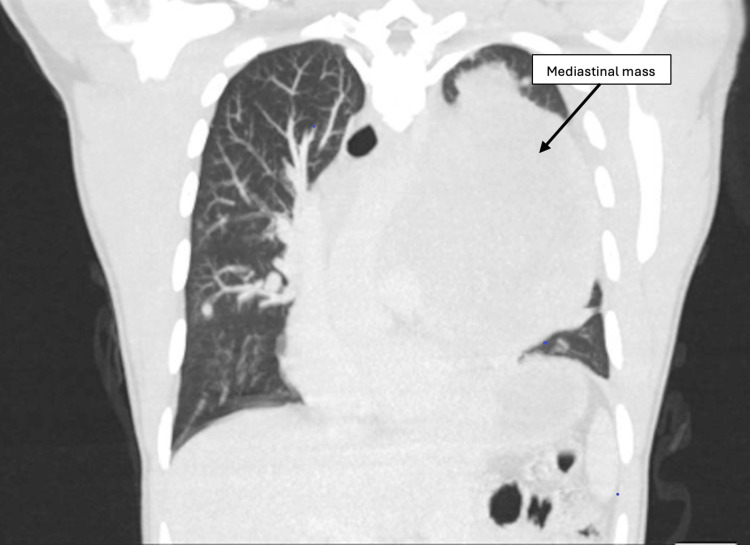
Coronal contrast-enhanced CT thorax demonstrating a large heterogeneous anterior mediastinal mass occupying much of the left hemithorax, with marked compression of the left lung and rightward mediastinal shift The mass shows contact with the pleura and chest wall. The black arrow indicates the anterior mediastinal mass.
